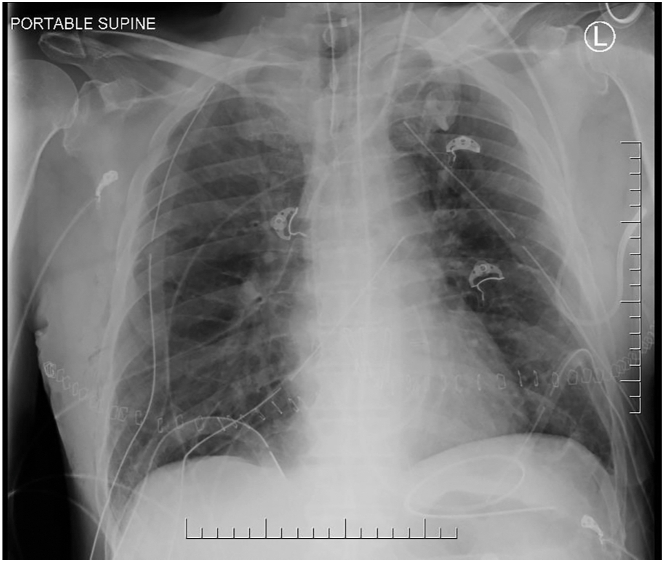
Chest radiograph of recipient upon arrival to the intensive care unit.
